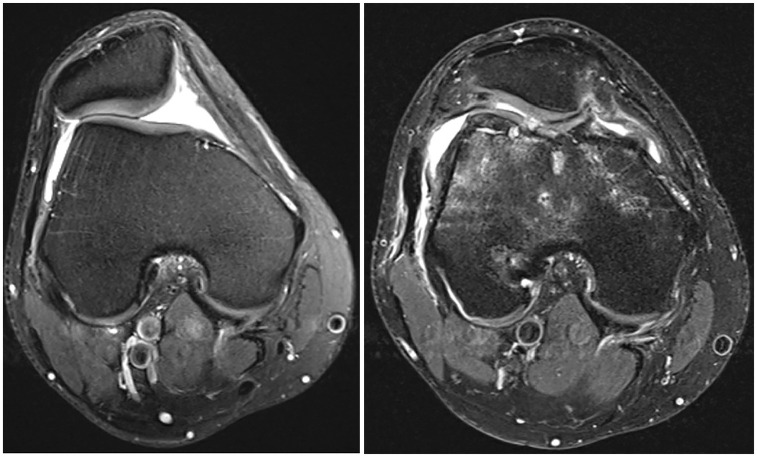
Preoperative and postoperative (at 22 months) axial magnetic resonance imaging of the patellofemoral joint in a patient who underwent medial patellofemoral ligament reconstruction, tibial tubercle osteotomy, lateral lengthening, and trochleoplasty with the progression of trochlear cartilage damage from grade 0 preoperatively to grade 4 postoperatively.
